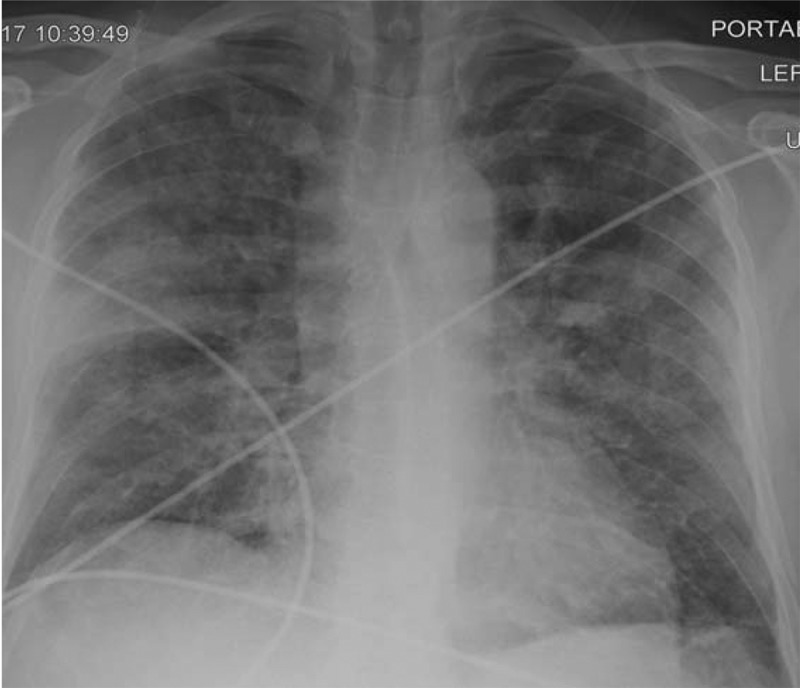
Chest radiograph on admission demonstrating bilateral diffuse multifocal infiltrates, most pronounced on the right side.
